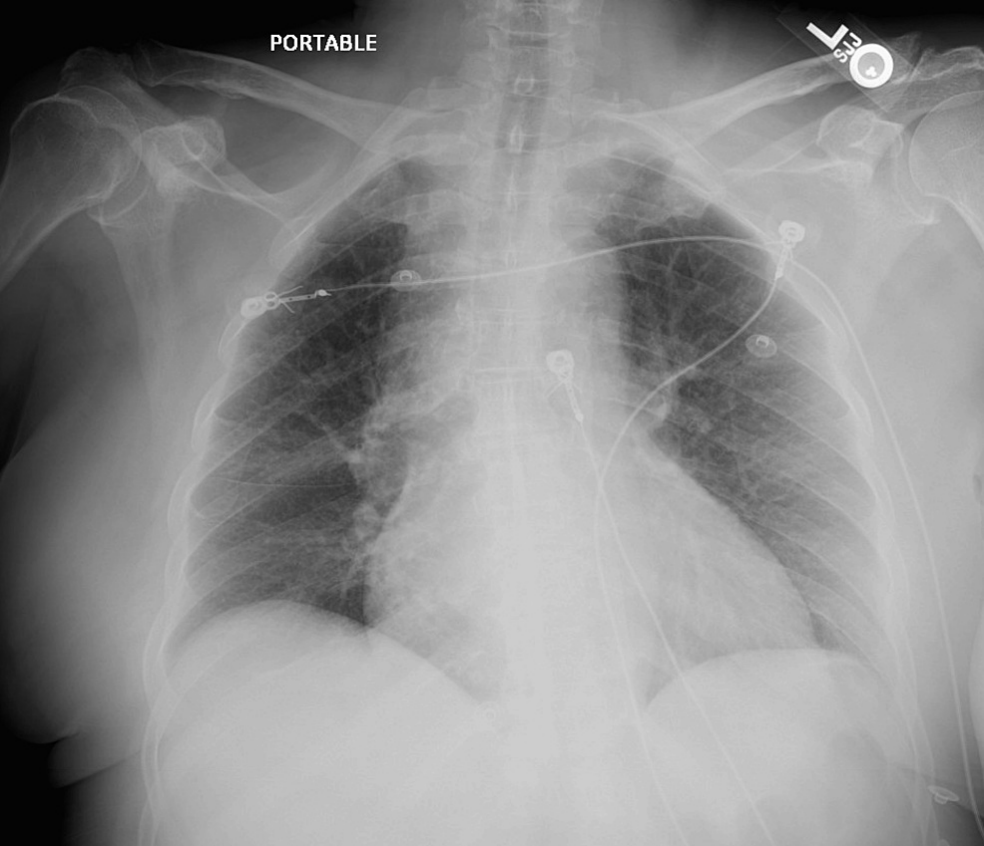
Chest X-ray (AP view) on the day of admission. Mild enlargement of the cardiac silhouette.
